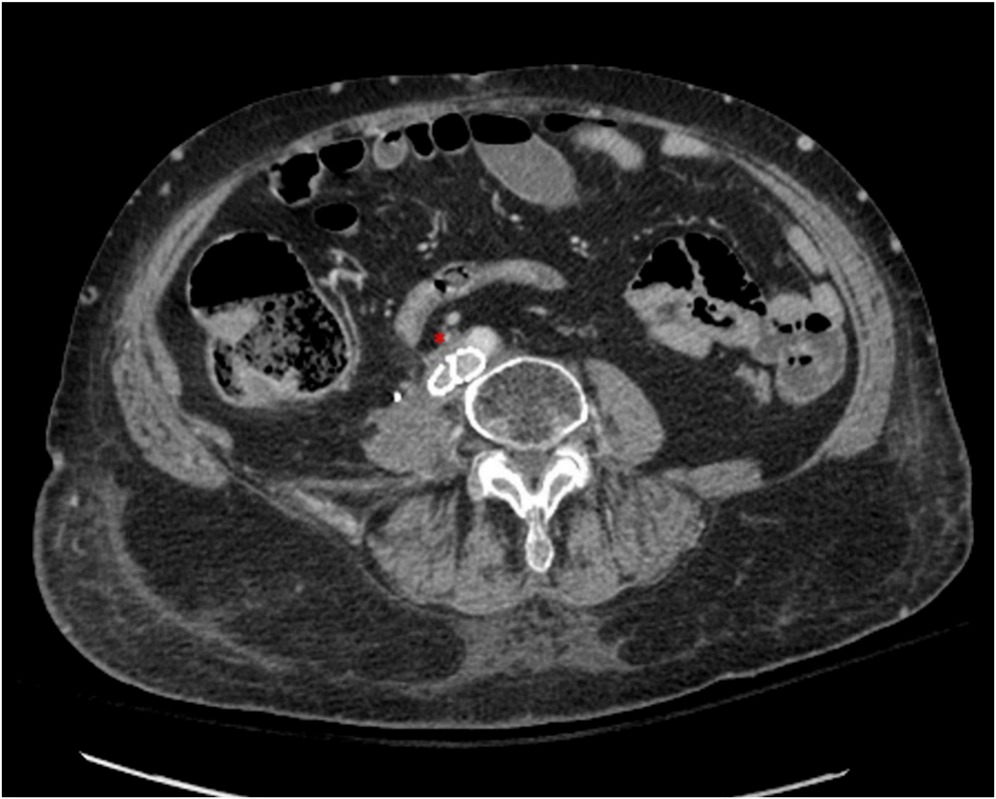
Axial CTA in venous phase shows compression of the right external iliac artery by the stent, and narrowing of the stent.
